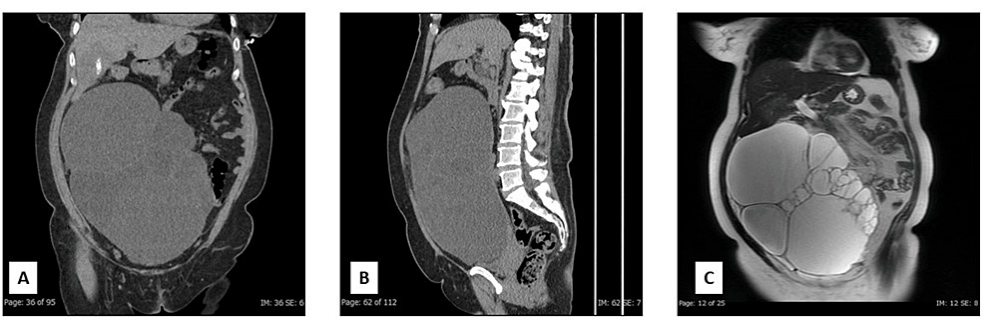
Abdominal CT scan, whole-body PET scan, and MRI of the patient. (A) Coronal image of the abdomen and pelvis without contrast demonstrates a complex multiseptated cystic mass of up to 24.6 x 18.1 x 11 cm arising from the right adnexa. An ill-defined 7.1 x 5.5 x 5.1 cm low-density lesion in the right hepatic lobe shows central calcifications. (B) A sagittal image of the abdomen and pelvis without contrast redemonstrates a complex multiseptated cyst mass, 24.6 cm in craniocaudal dimension and arising from the right adnexa. (C) Coronal T2 Single-Shot Fast Spin Echo (SSFSE) ARC image of the abdomen and pelvis shows a large 26 x 19 x 13 cm multiloculated cystic structure that appears to originate from the right adnexal region and demonstrates T2 hyperintense signal with multiple thickened septations.
